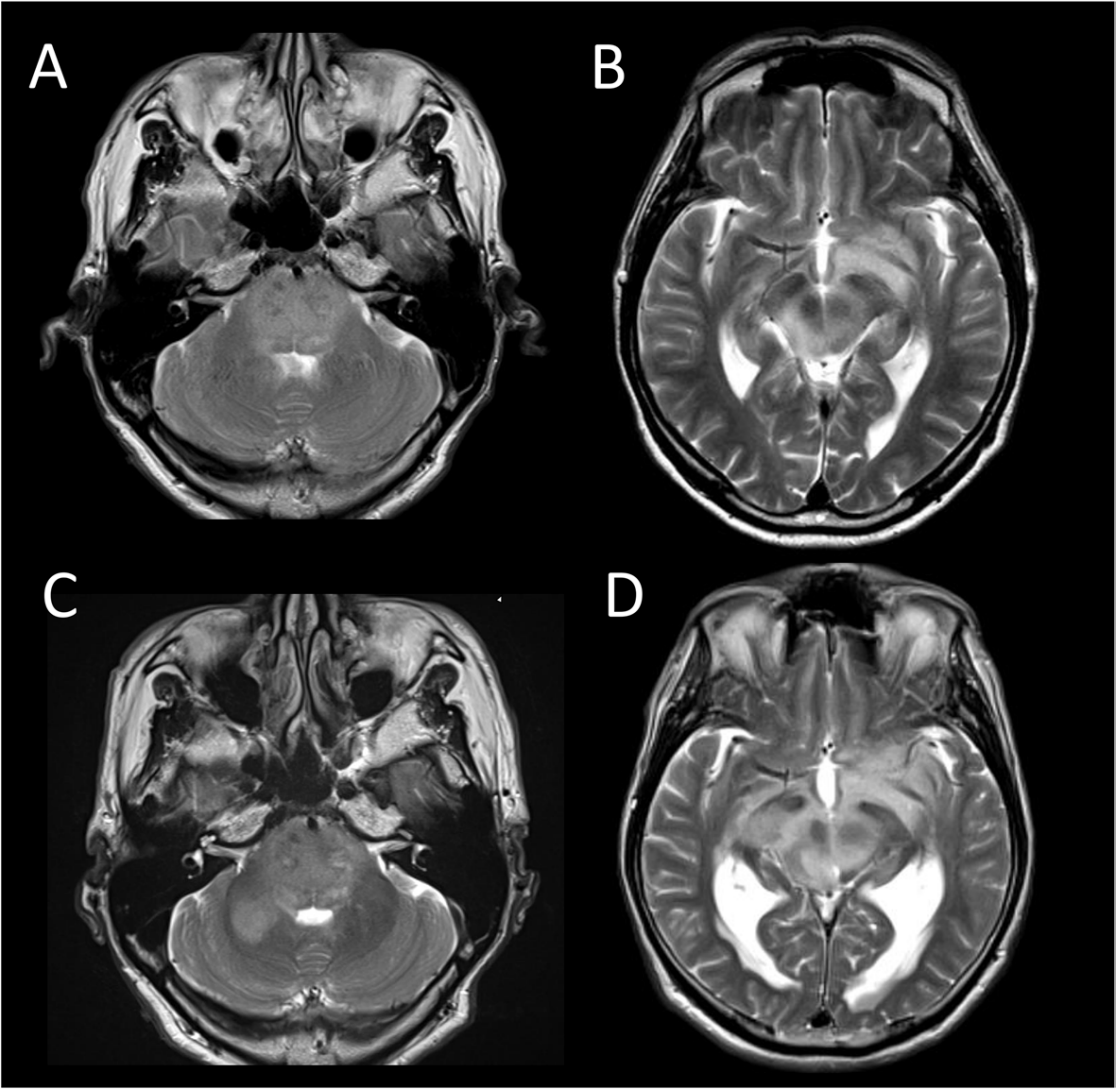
The patient presented with high-intensity lesions on T2-weighted imaging of the pons (A). The lesion extended to the midbrain (B). The patient initially preferred to be followed-up at an outpatient clinic, during which time the lesion extended to the right middle cerebellar peduncle (C) and involved the midbrain and thalamus bilaterally (D)
